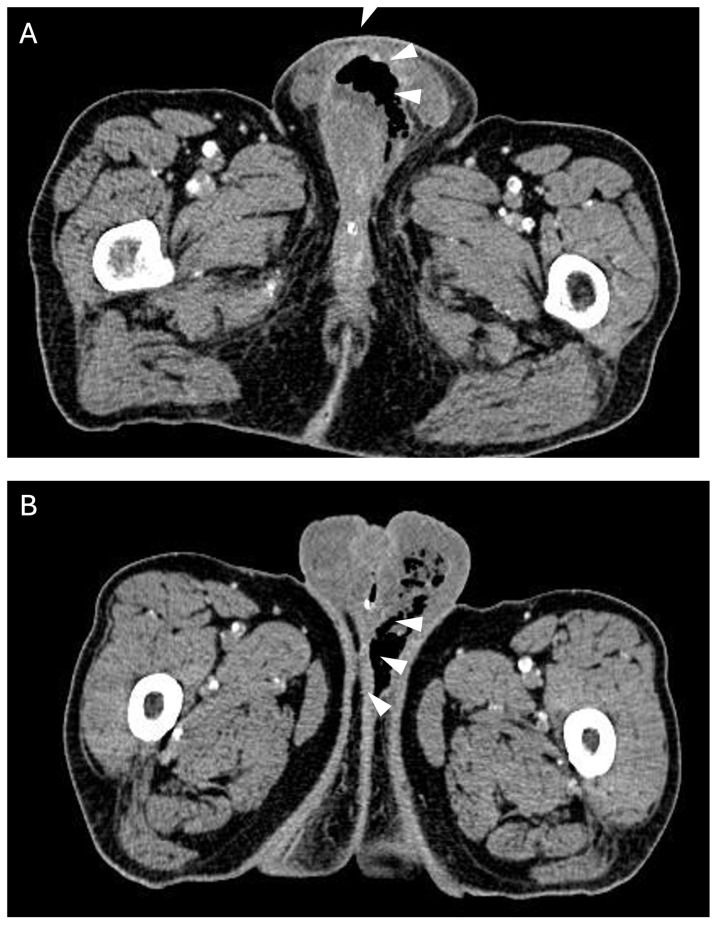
Axial CT images (A,B) show extensive soft tissue stranding with edema in penis and scrotum and a large volume of gas air (arrowheads) tracking into deep soft tissues of perineum representing Fournier gangrene.
